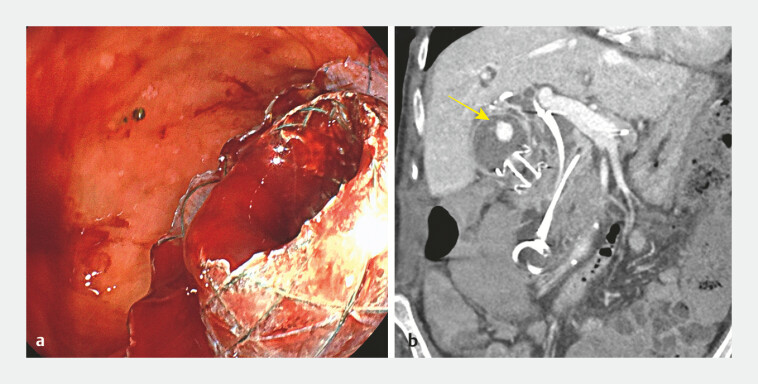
Endoscopic and radiologic findings of pseudoaneurysm following endoscopic ultrasound-guided gallbladder drainage.
